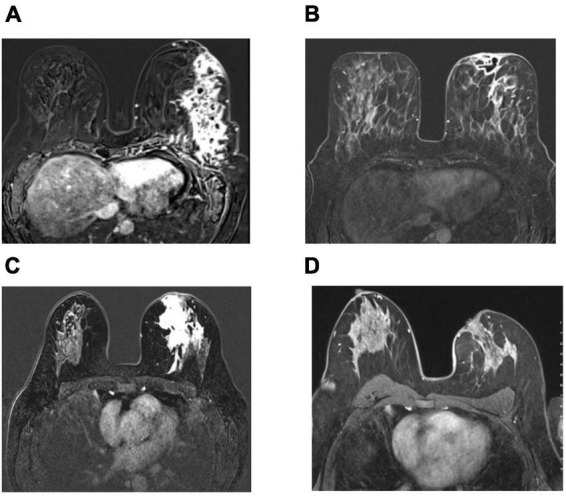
Axial pretreatment contrast enhanced images (A,C) show contrast enhancement at the inflamed area extending to the prepektoral region with the collection area that opened to the skin (A), and contrast enhancement at the inflamed area extending to the periareolar region (C). Complete radiological response after therapy in 2 patients treated with local steroid injection and oral low-dose steroid use as complete disappearance of contrast enhancement in subtraction images in MRI (B,D).
